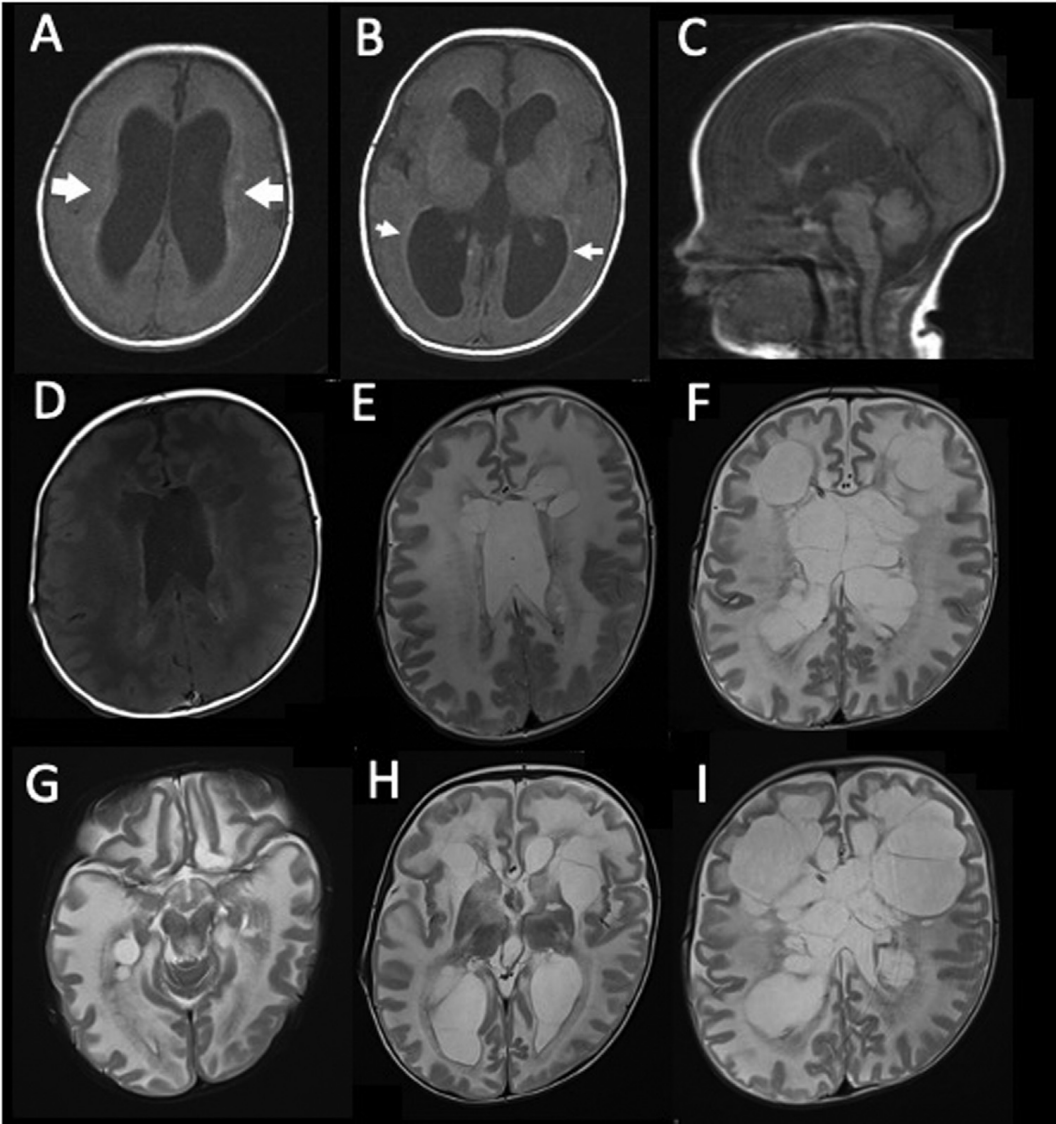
Magnetic resonance imaging of the brain. (A–C), T1-weighted images 2 days after birth. (A), the lateral ventricles are enlarged, and a hyperintense signal in the deep white matter is observed (large white arrow). (B), a hyperintense signal classified as periventricular rim is shown (small white arrow). (C), the sagittal image shows a narrow aqueductus cerebri. (D and E), images at 5 months of age. (D), the T1-weighted image shows a hyperintense signal in the deep white matter and cavitation localized to the frontal area. (E), the T2-weighted image shows a diffuse hyperintense signal in the white matter. (F–H), T2-weighted images at 10 months of age. (F), cystic transformation of the white matter extends to the occipital area. (G), a hyperintense signal in the brainstem is shown. (H), atrophy of the thalamus is observed. (I), the T2-weighted image at 13 months shows further extension of the cavitation
